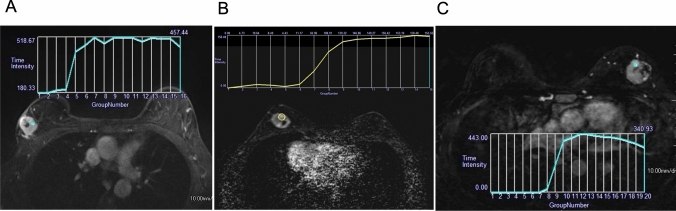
Comparison of time–intensity curves (TIC) across different ultrafast dynamic contrast-enhanced MRI techniques. The upslope of the TIC from the k-space-weighted image contrast (KWIC, time resolution: 3.8 s) is slightly jagged (A) compared to a time-resolved angiography with interleaved stochastic trajectories-volumetric interpolated breath-hold examination (VIBE) acquisition with a temporal resolution of 4.6 s (B), and compressed sensing-VIBE with a temporal resolution of 3.7 s (C). All mass lesions show rim enhancement and relatively steep upslopes indicative of malignancy. Pathology results showed invasive ductal carcinoma (A and B) and encapsulated papillary carcinoma (C), respectively
